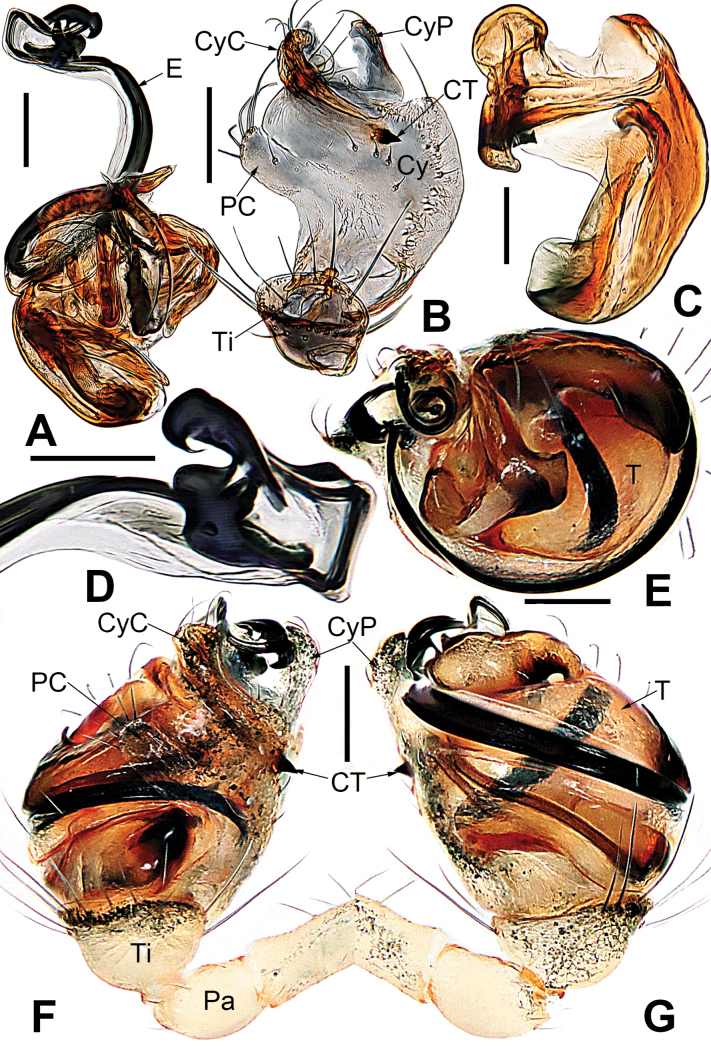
Microdipoenajobi* Kraus, 1967 from Georgia A bulbus with conductor removed, from behind B cymbium, prolateral C conductor, dorsal D embolic end, retrolateral E male palp, apical F male palp, prolateral G male palp, retrolateral. Abbreviations: CT = cymbial tooth, Cy = cymbium, CyC = cymbial conductor, CyP = cymbial process, E = embolus, Pa = patella, PC = paracymbium, T = tegulum, Ti = tibia. Scale bars: 0.10 mm.
